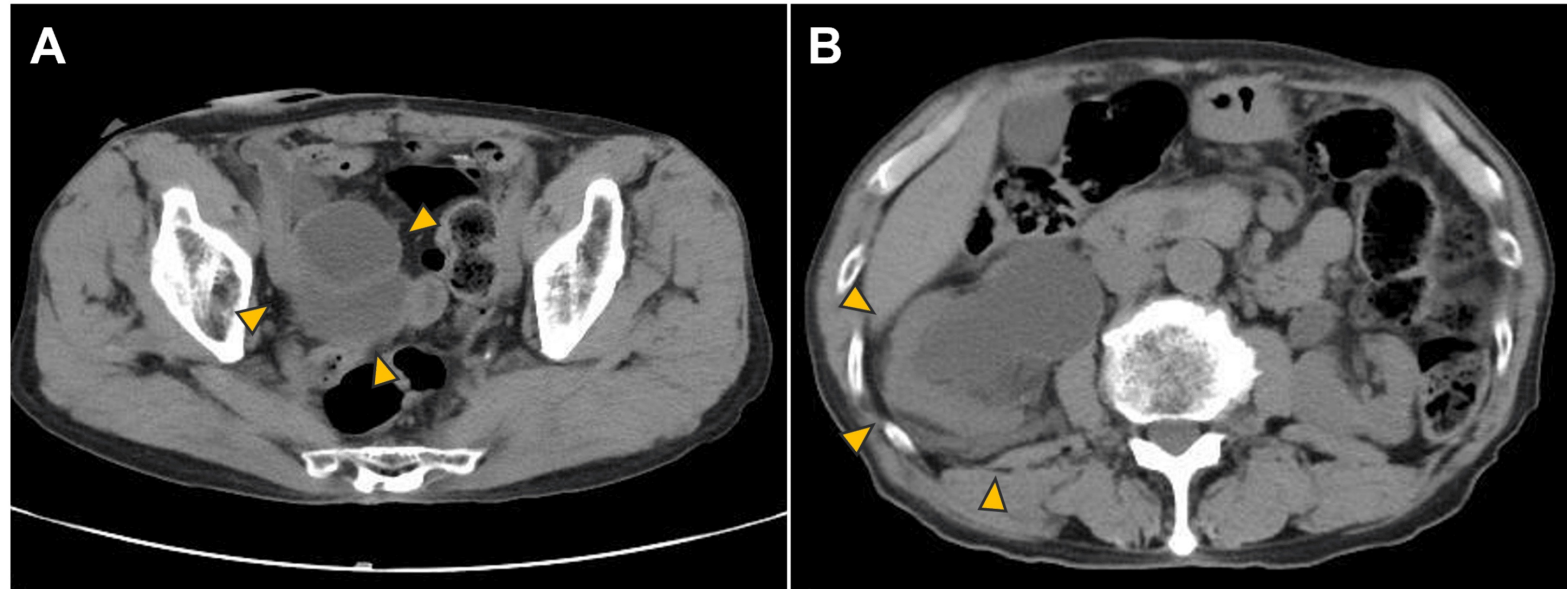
Computed tomography (CT) findings at the onset of symptoms (three years prior) Axial CT images were obtained three years prior to the current presentation. (A) Image showing the dilated ileal conduit (orange arrowheads). (B) Image demonstrating right hydronephrosis (orange arrowheads). Although these findings indicated urinary tract obstruction, the definitive cause was not identified at this stage.
